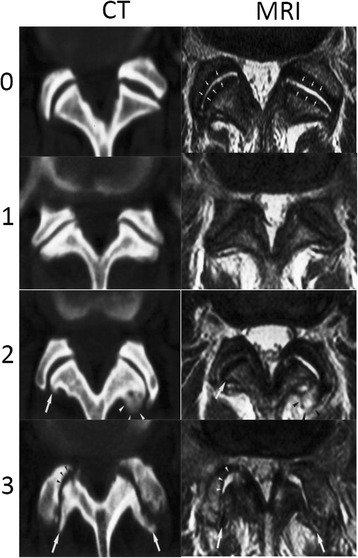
The Weishaupt grade of CT and MRI images of the lumbar facet joint: Grade 0, normal facet joint space (2–4 mm width); Grade 1, narrowing of the facet joint space (<2 mm) and/or small osteophytes, and/or mild hypertrophy of the articular process; Grade 2, narrowing of the facet joint space and/or moderate osteophytes, and/or moderate hypertrophy of the articular process, and/or mild subarticular bone erosions; and Grade 3, narrowing of the facet joint space and/or large osteophytes, and/or severe hypertrophy of the articular process, and/or severe subarticular bone erosions, and/or subchondral cysts
